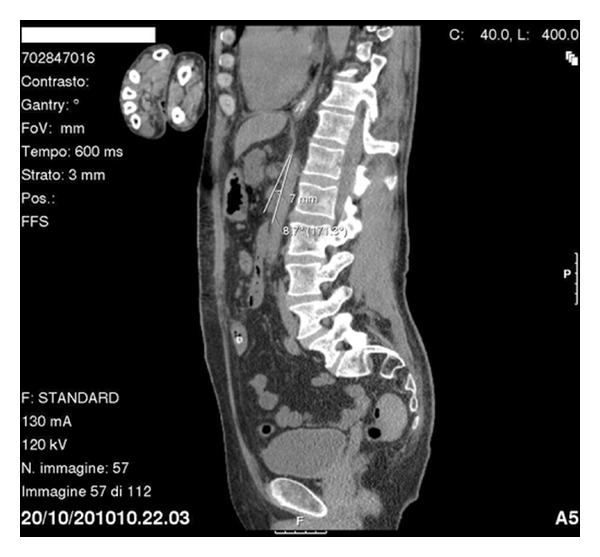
Sagittal CT image demonstrating the aortomesenteric angle.
